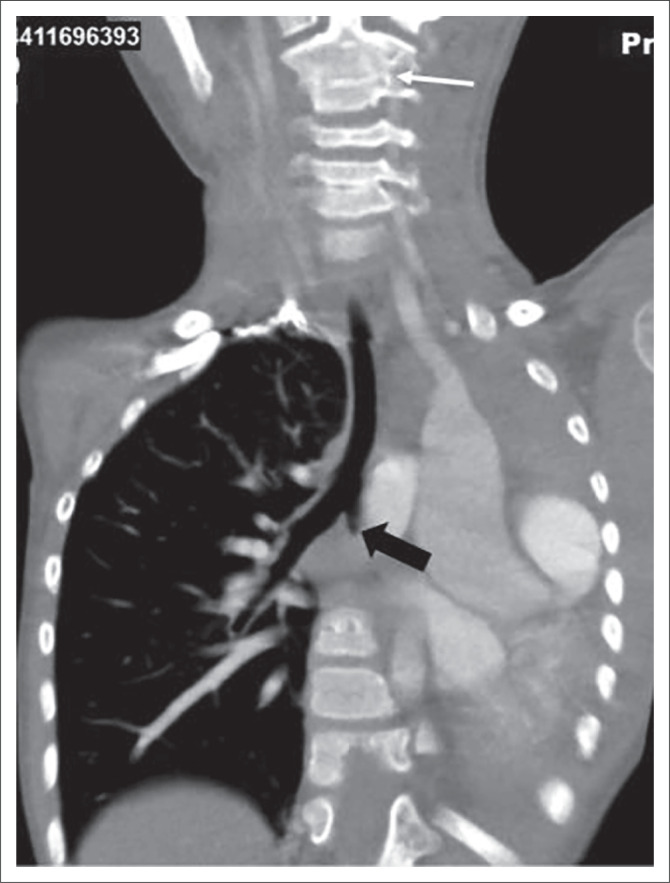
Coronal Computed tomography angiography thorax demonstrates absence of the left lung and pulmonary vessels. The LMB shows abrupt termination after the carina (thick black arrow). The mediastinum is completely displaced to the left side. There is associated fusion of the C2–C3 vertebrae (thin white arrow).
